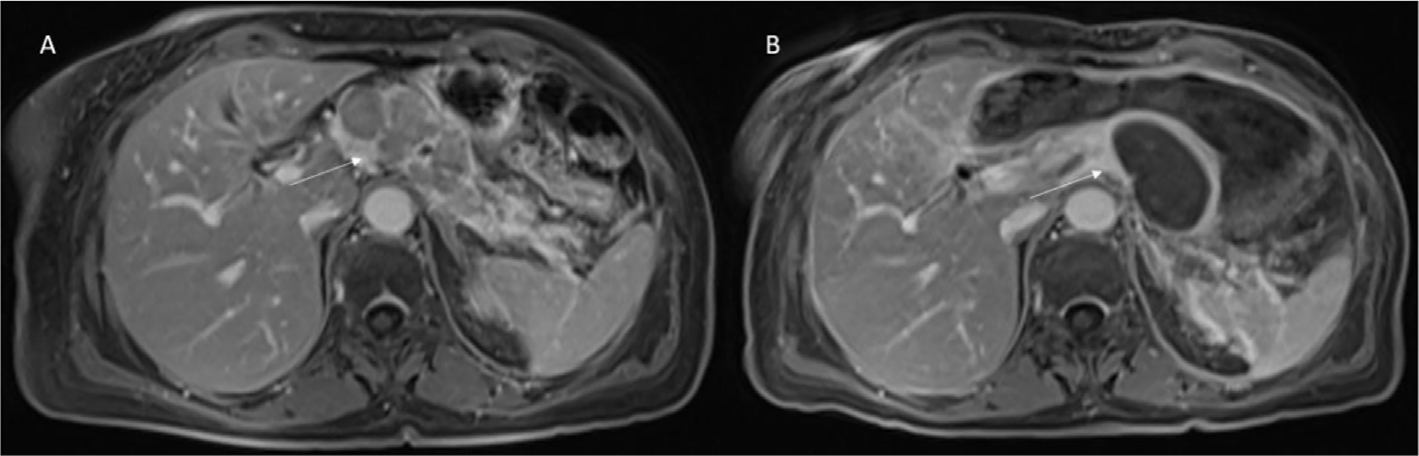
Patient 1 with Body-Tail Pancreatic Cancer. Morphological MRI assessment post-RFA treatment. In (A) (VIBE T1-W post-contrast sequence during portal phase in axial plane) pre-treatment evaluation of lesion (arrow). In (B) (VIBE T1-W post-contrast sequence during portal phase in axial plane) arrow shows ablated area. Qualitative assessment shows significant differences in SI in pre- and post-treatment sequences.
